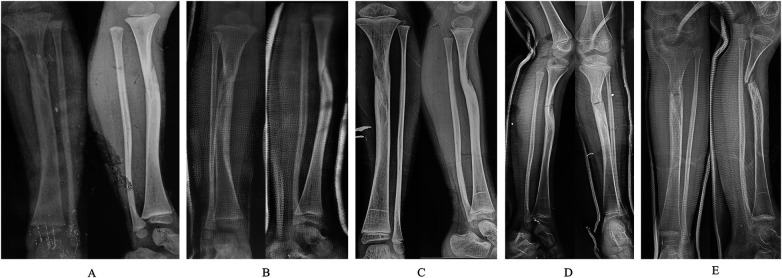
Serial radiographs of the left tibia and fibula before hospitalization. (A) On June 7, 2020, radiographs showed initial fracture when the patient was 3 years and 10 months old. (B) On July 22, 2020, proximal tibial dysplasia was obvious. (C) In January 2022, radiographs demonstrated incomplete fracture union. (D) Refracture occurred three months before admission. (E) Radiographs 1 month prior to admission showed non-union and pseudarthrosis formation.
